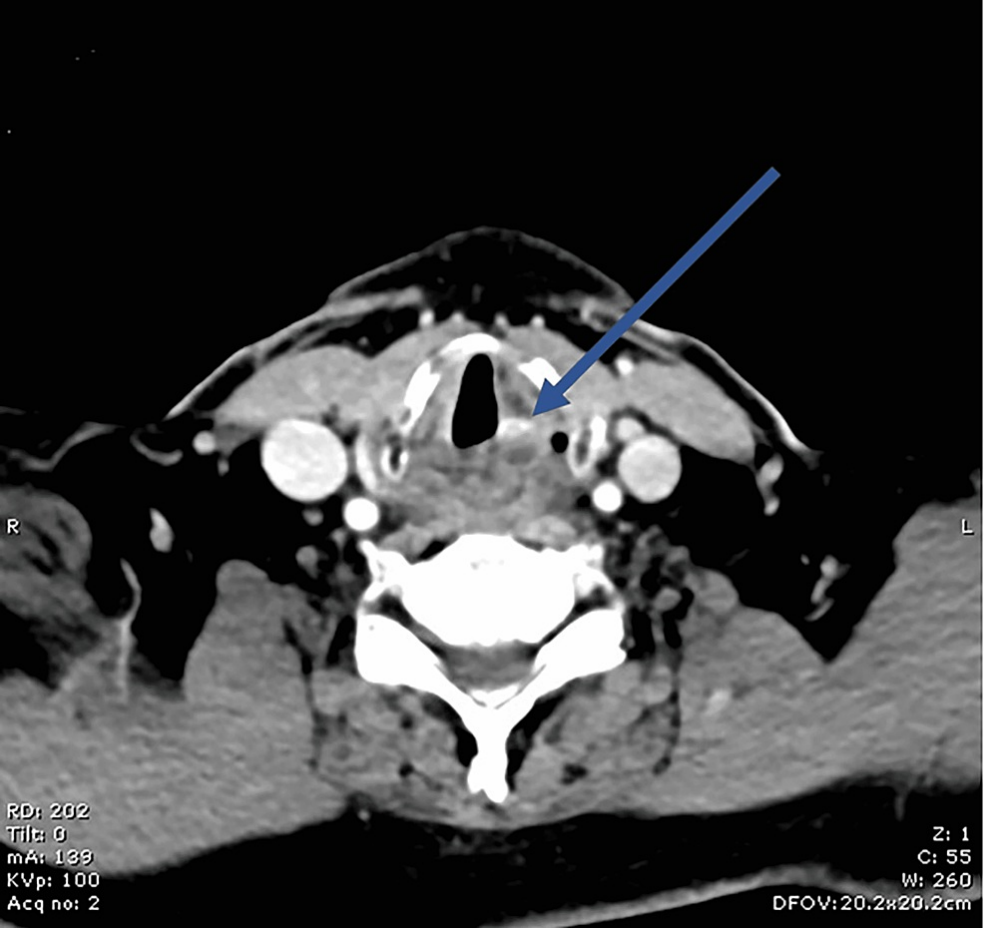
CT neck with contrast image at C6 level showing high-density foci within the post-cricoid region (arrow)
